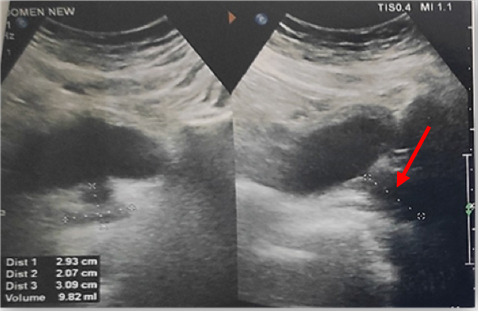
Liver ultrasound images showed a hypoechoic focal area with irregular outline (red arrow, an abscess cavity), but there was no demonstrable foreign body.
